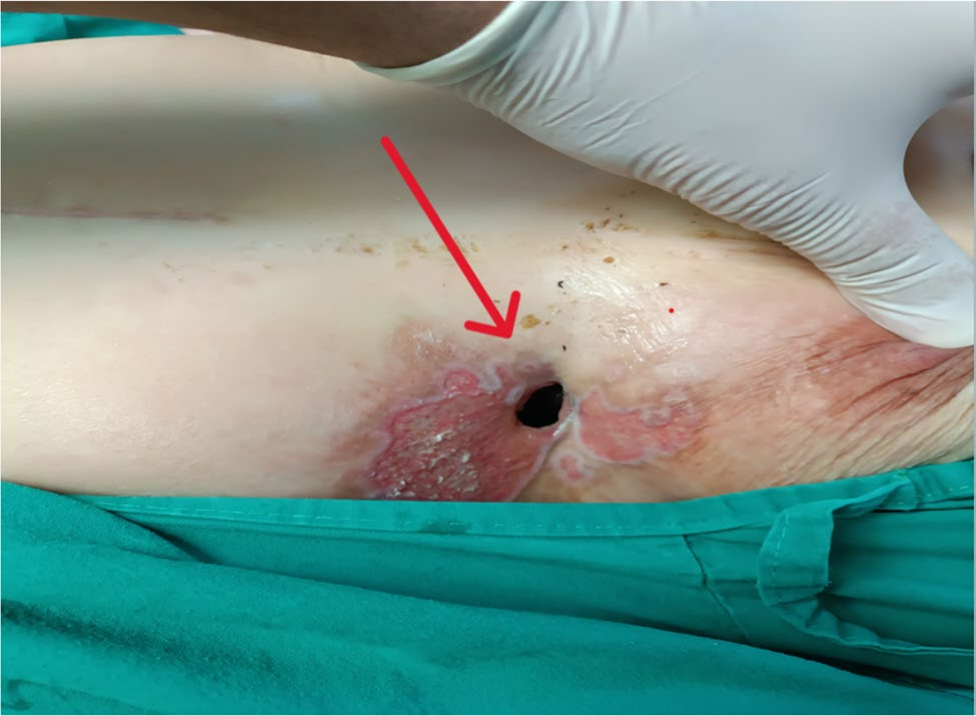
Oblique 3D CT reconstruction emphasizing the spatial relationship between the cement prosthesis (red arrow), missing ribs, and remaining thoracic cage. The prosthesis appears adherent to surrounding structures with evidence of chronic structural deformation
